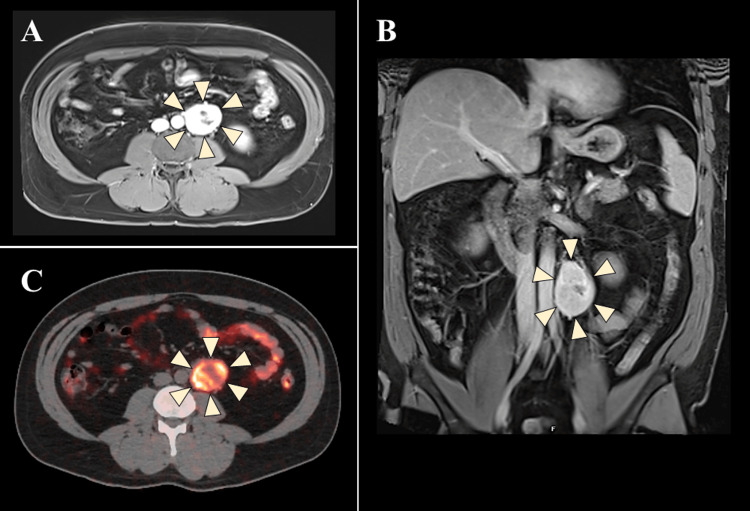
Patient 2 - MRI of the abdomen (A, B) and copper-64 Dotatate PET-CT scan (C) Yellow arrowheads frame the margins of the mass. MRI, magnetic resonance imaging; PET-CT, positron emission tomography-computed tomography
